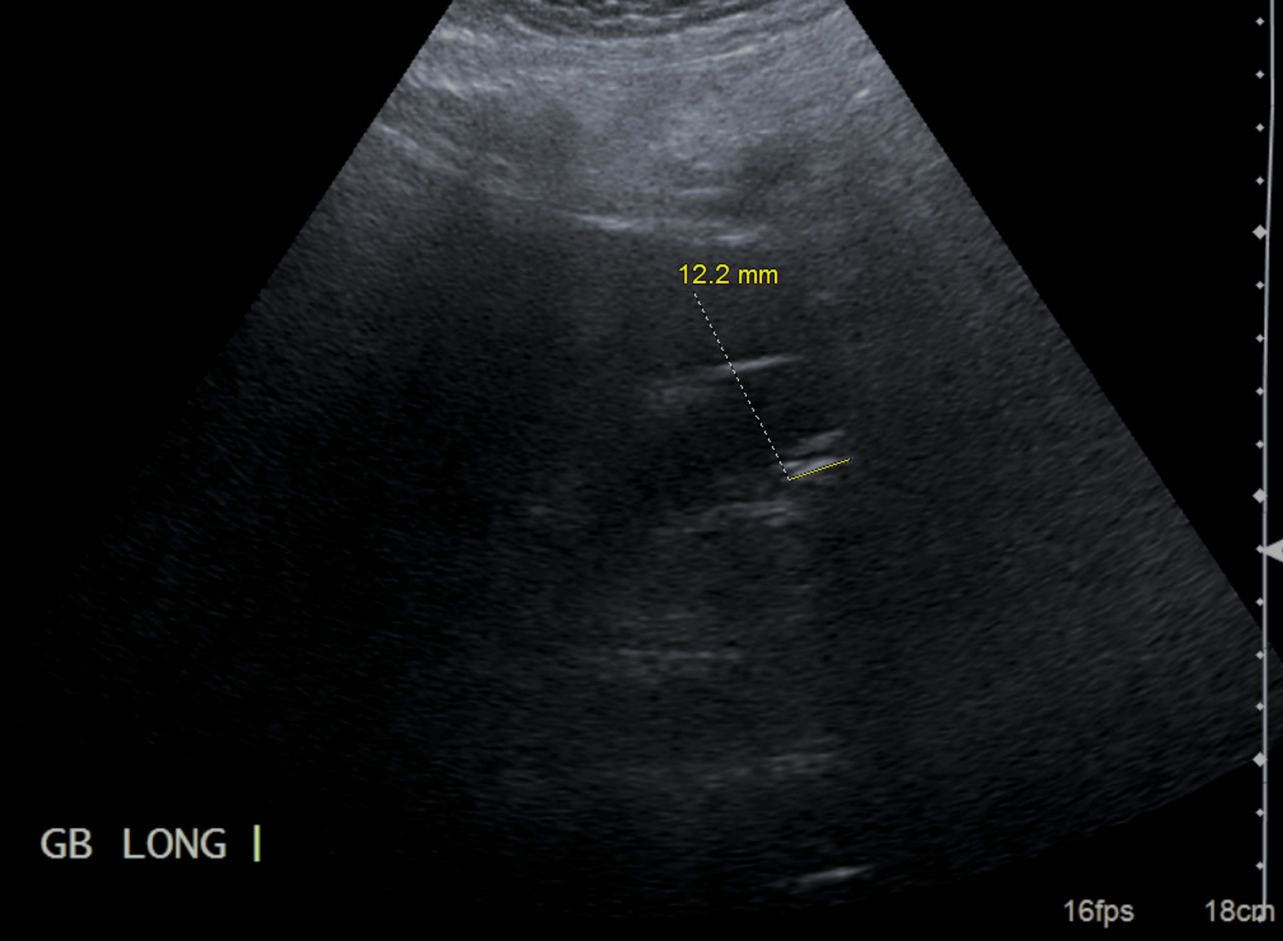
Ultrasound of the right upper quadrant measuring a normal gallbladder length and without findings of gallbladder wall thickening or pericholecystic fluid. The yellow line indicates the length of the gallbladder.
